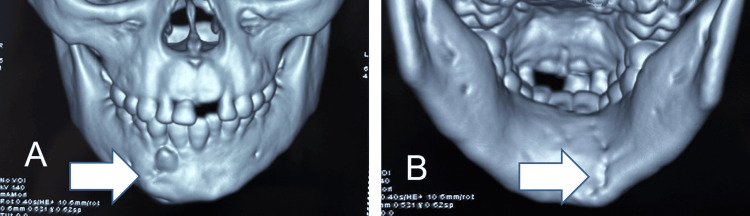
Postoperative CT scan A: frontal view, B: lingual view Arrow depicts the healed fracture site
